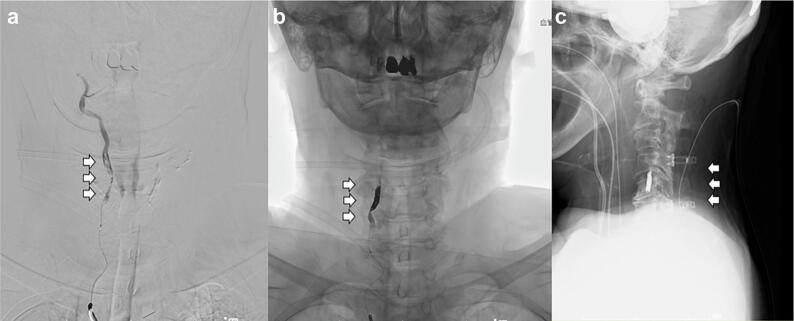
Postoperative radiographic images and coil embolization. (a) Intraoperative angiographic image taken during coil embolization showing irregular contrast opacification at the C4–C7 levels, suggestive of intimal injury to the vertebral artery (arrow). (b) Post-embolization angiogram confirming complete occlusion of the left vertebral artery (arrow). (c) Postoperative lateral radiograph demonstrating stable spinal alignment following C4–C7 laminoplasty (arrow).
